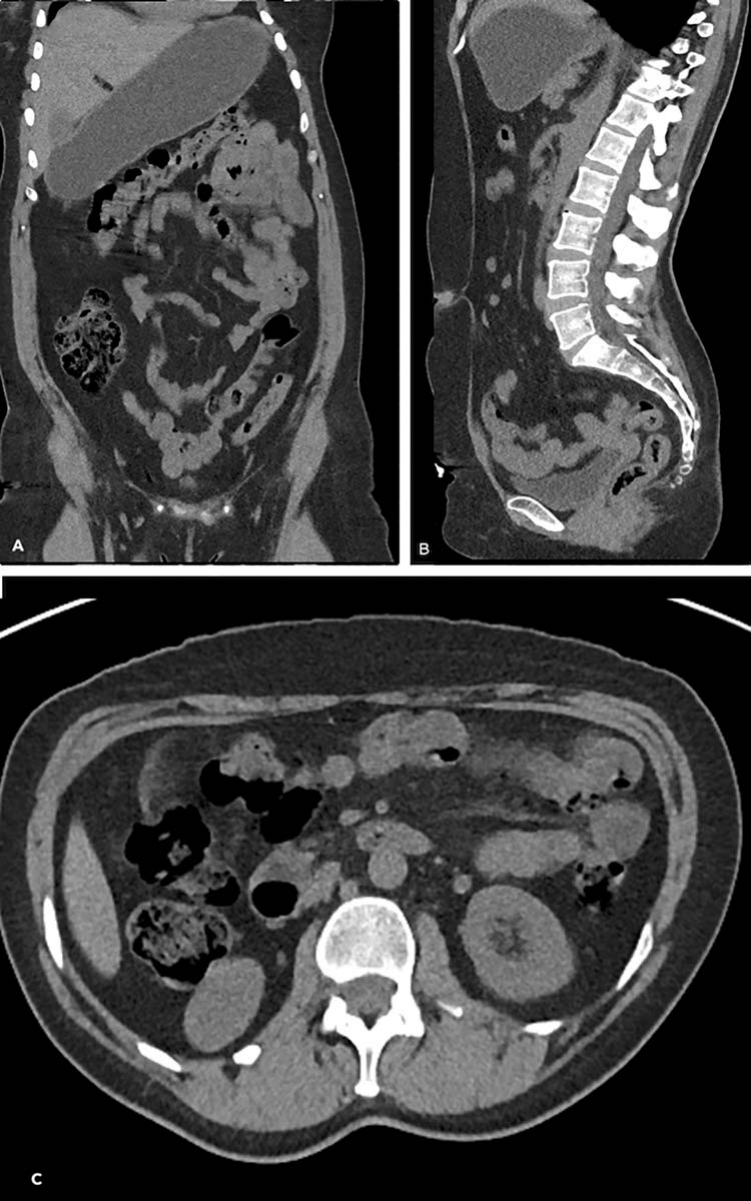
The CT scan abdomen that patient had 6 months prior to the presentation to the ED with renal colic. (A) Coronal, (B) sagittal and (C) axial. There is no evidence of tumour in the transverse colon.
